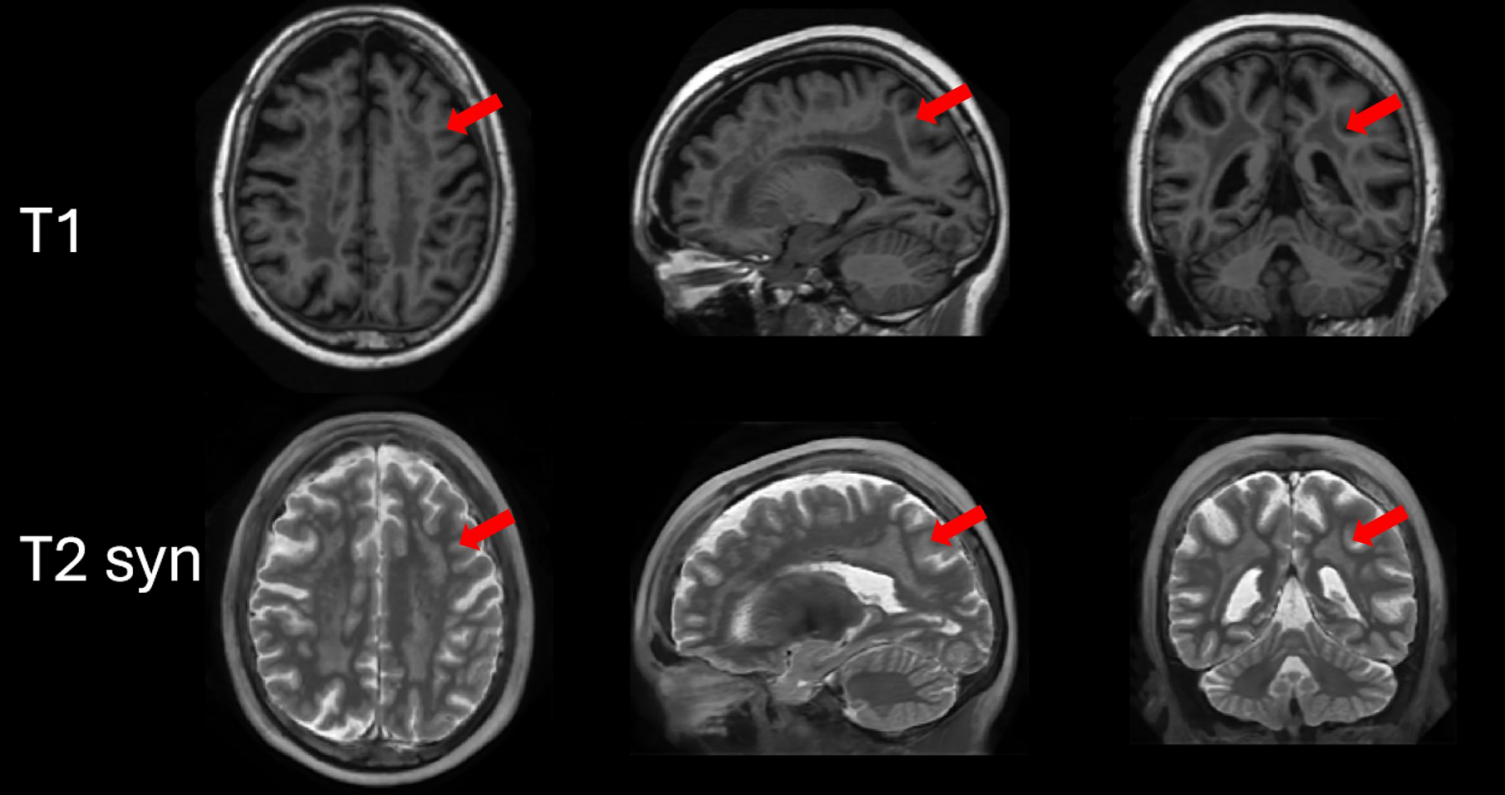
Qualitative results on the OASIS dataset. Top row: T1-weighted input images with visible white matter confluent lesions. Bottom row: synthesized T2-weighted images produced by the proposed method, showing consistent lesion depiction (red arrows).
